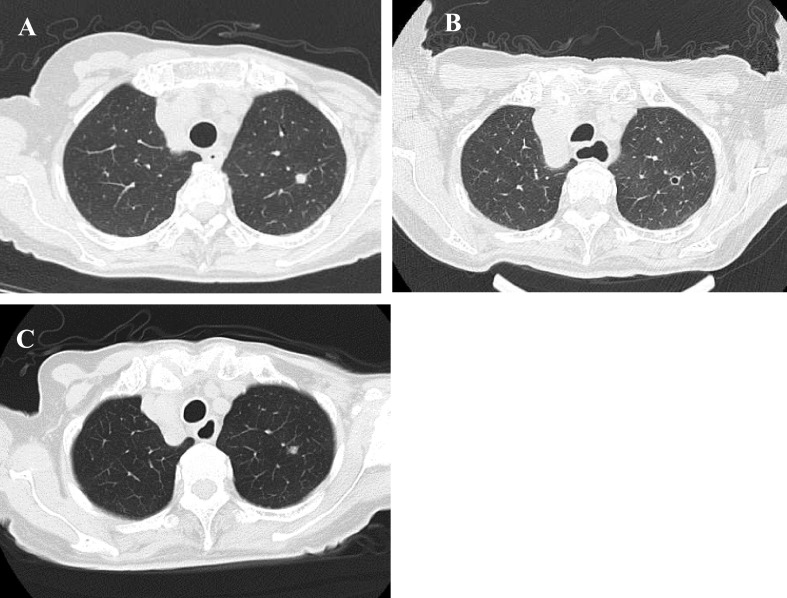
CT scans of patient’s chest. A: First day of hospitalization. B:
